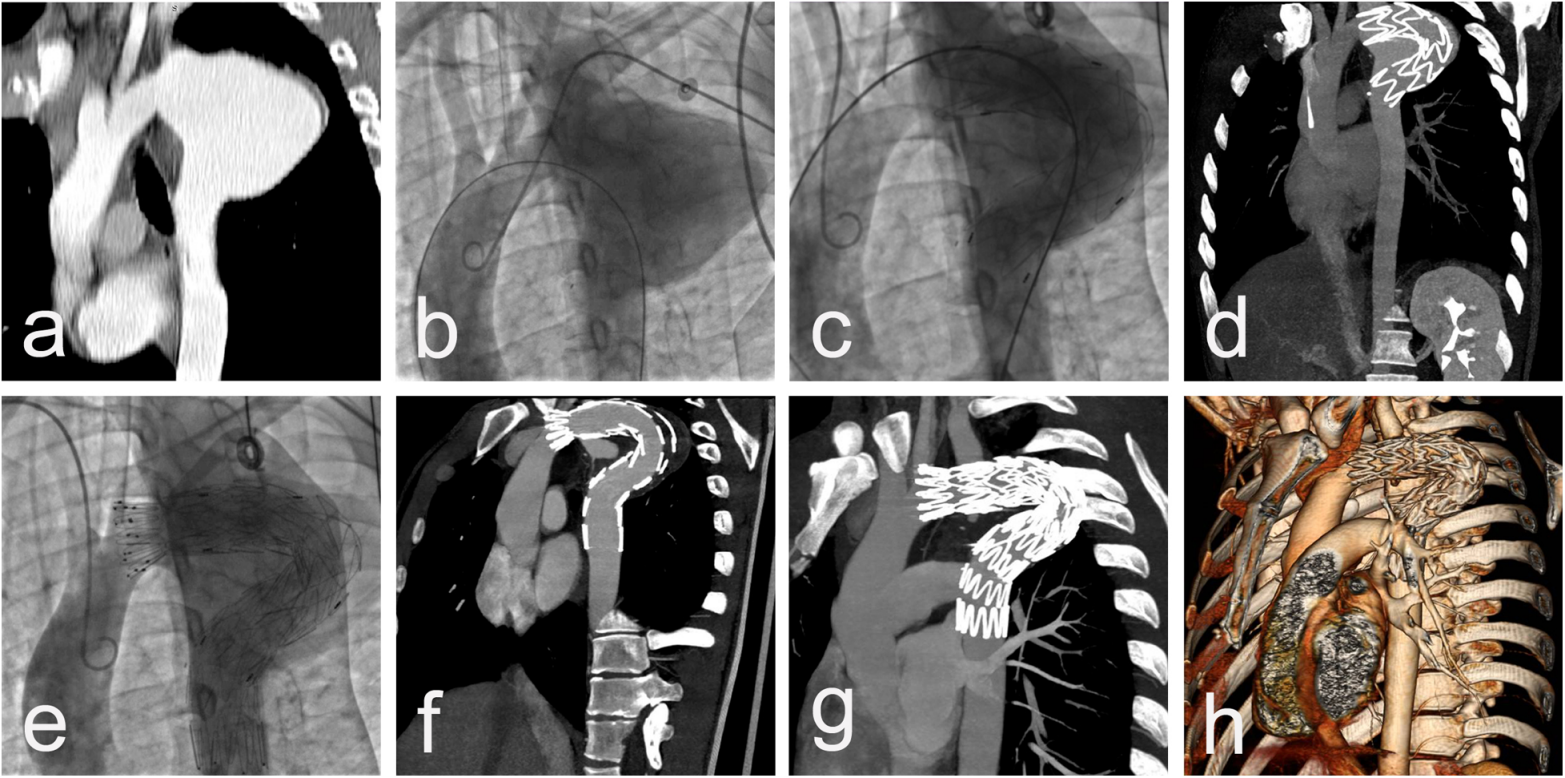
CT scan imaging (a) and aortic angiography (b) demonstrating saccular thoracic aortic aneurysm engaging LSA of 27 y/o male presenting 15 years after initial surgical repair with cough and hemoptysis. Dimensions of aneurysm 56/70 mm with no signs of thrombosis. Postdelopyment aortography revealing a collapse of the stent-graft in the large aneurysm, accepting its large curvature with severe type I endoleak (c), confirmed by immediately performed CTA, with no sign of iatrogenic rupture and preserved distal blood flow (d) Additional stent-graft positioned right behind left carotid artery and complete aneurysm isolation (e) with post-procedure CT data of completely covered LSA and small endoleak type II from large LSA (f) CT scan imaging at 1-year follow-up with complete aneurysm thrombosis, reduction of the aneurysm size with 40% and lack of endoleaks (g,h).
